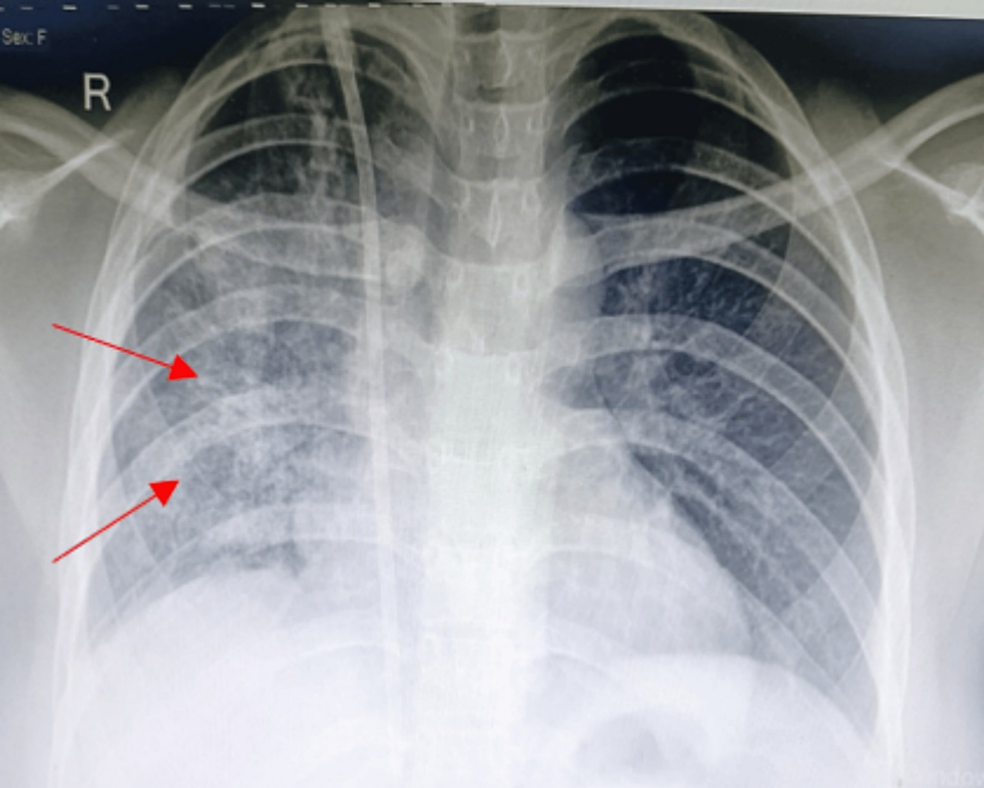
Chest X-ray showing resolving lung infiltrates after treatment (red arrows)
